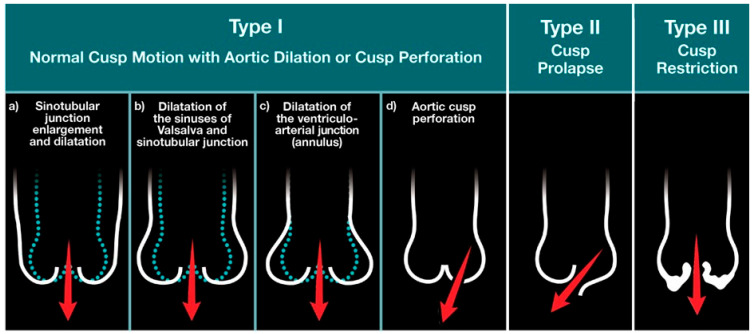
Carpentier Classification of Etiologies of Aortic Regurgitation. Aortic regurgitation can be classified by the etiology of aortic regurgitation as: leaflet malcoaptation/perforation (Type I), excessive leaflet motion (Type II), or restricted leaflet motion (Type III). Type I disease can be further subclassified into the location of the aortic root dilatation: sinotubular junction, sinuses of Valsalva, or ventriculoarterial junction. (After Zoghbi WA, Adams D, Bonow RO, et al. Recommendations for Noninvasive Evaluation of Native Valvular Regurgitation: A Report from the American Society of Echocardiography Developed in Collaboration with the Society for Cardiovascular Magnetic Resonance. *J Am Soc Echocardiogr.* [10]).
